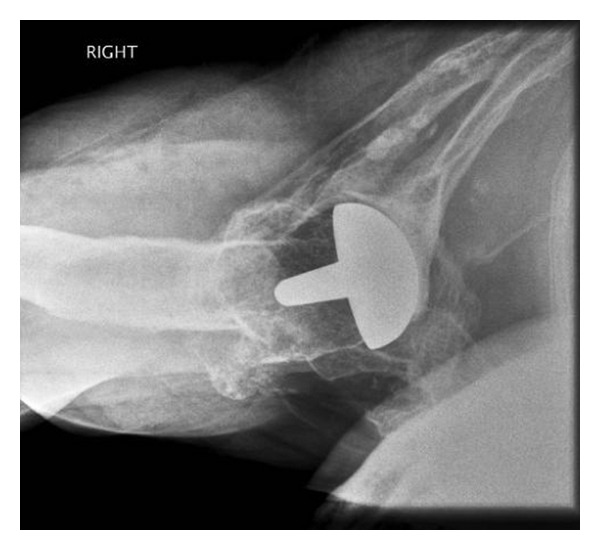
Axillary lateral radiograph of the right shoulder taken 4 years postoperatively.
